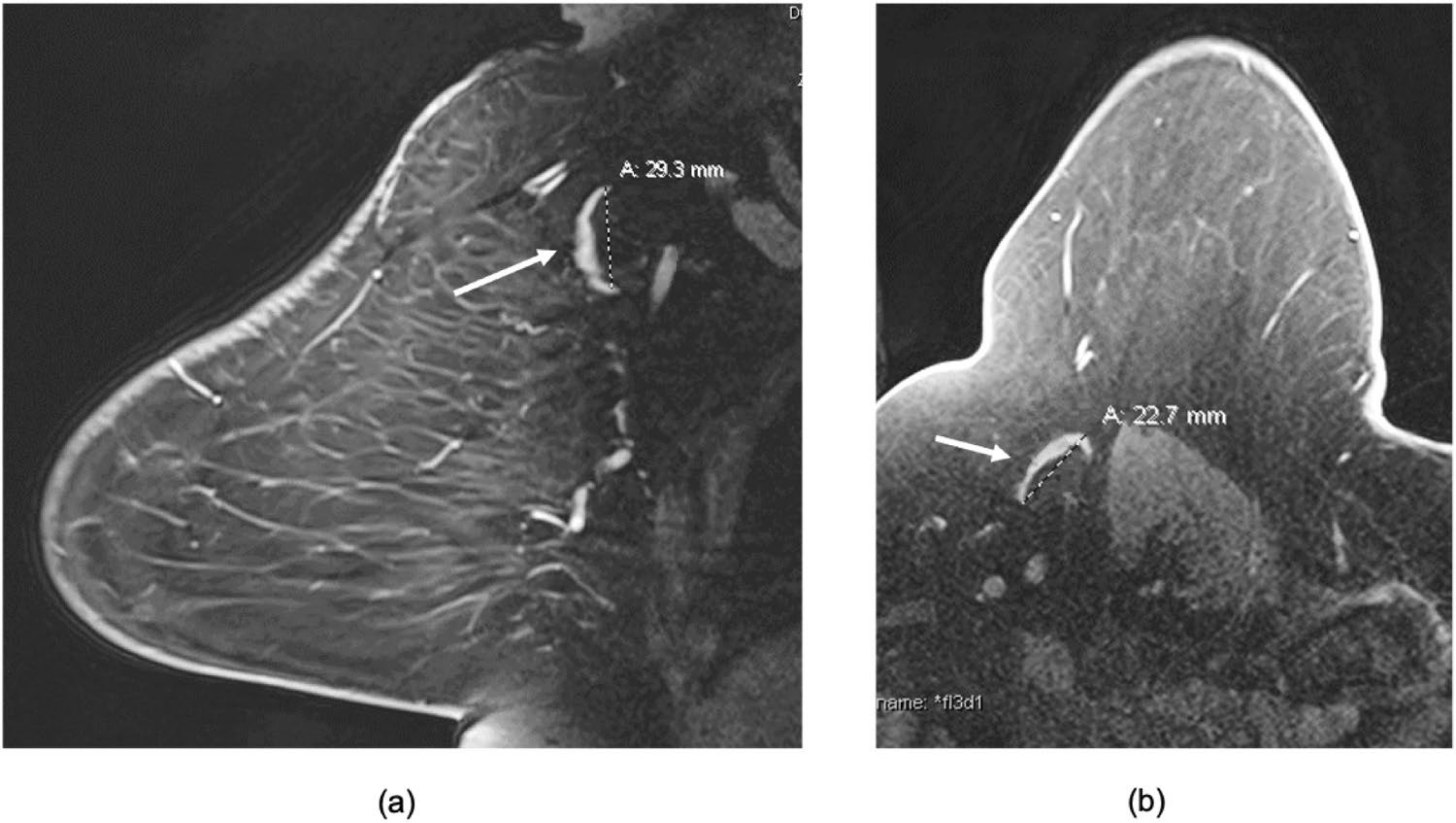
Axillary node measurements on breast MRI. 71 y/o female with left breast 18 mm ER+ HER2− node-positive invasive ductal cancer showing differences in the measurements of a single lymph node in the contralateral right axilla in the sagittal and axial plane. a Sagittal contrast enhanced fat-saturated breast MRI image through the contralateral right axilla demonstrates a fat-enlarged lymph node measuring 29 mm in greatest dimension. b Axial contrast enhanced image through the same index node shows smaller greatest dimension of 23 mm. We chose the largest measurement (in the sagittal or axial plane) for our study and in this case, we used 29 mm for the analysis as identified in the sagittal plane from (a)
